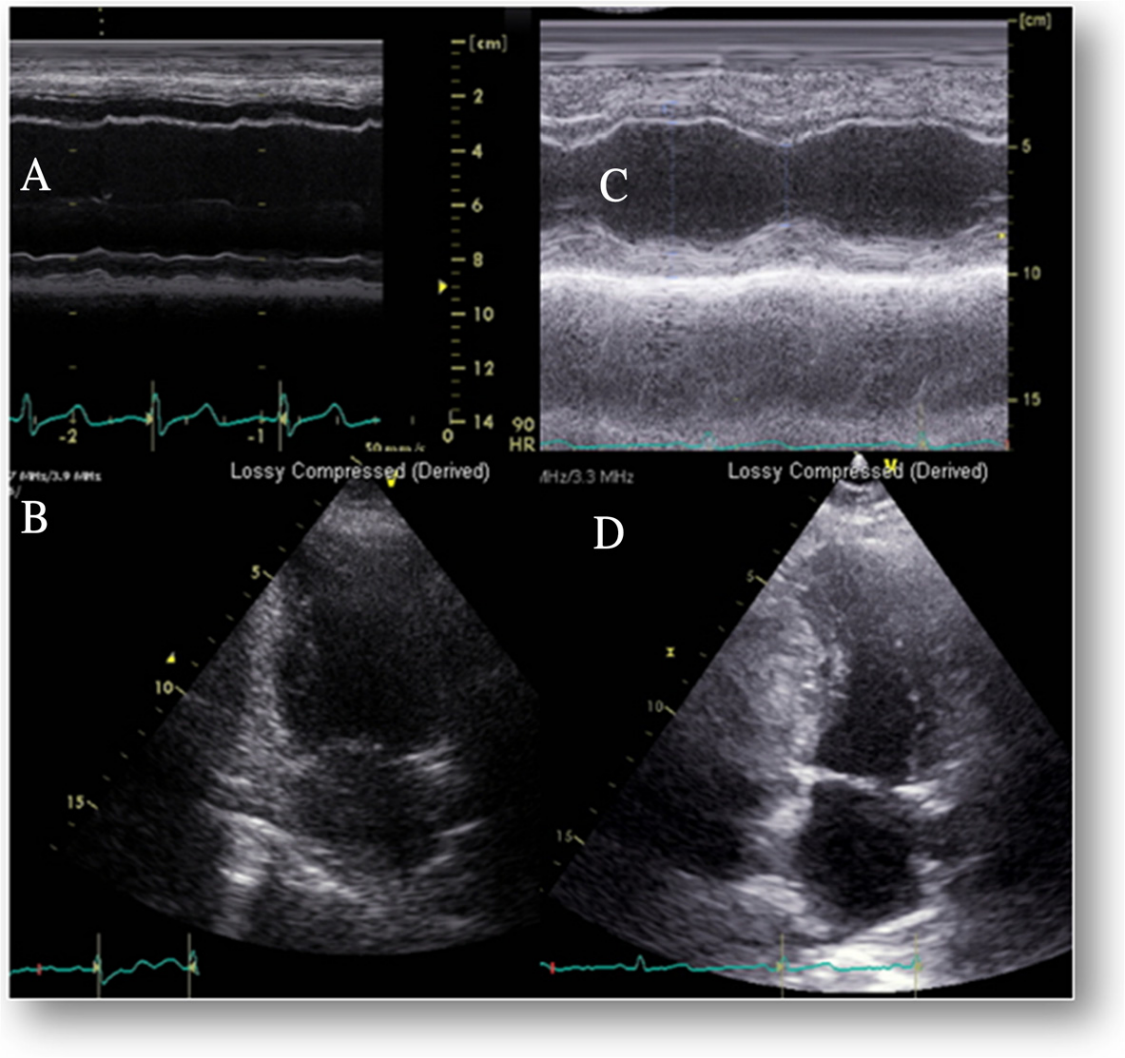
Echocardiographic findings on admission and early follow-up. a Admission, Basal MMode showing dilated left ventricle with severe systolic function. b Admission, end systolic apical 2-chambers view demonstrating large end systolic volume. c Follow-up at 3 weeks, Basal MMode showing normal ventricular size and contraction. d Follow-up at 3 weeks, end systolic apical 2-chambers view with normal ventricular size and contraction
